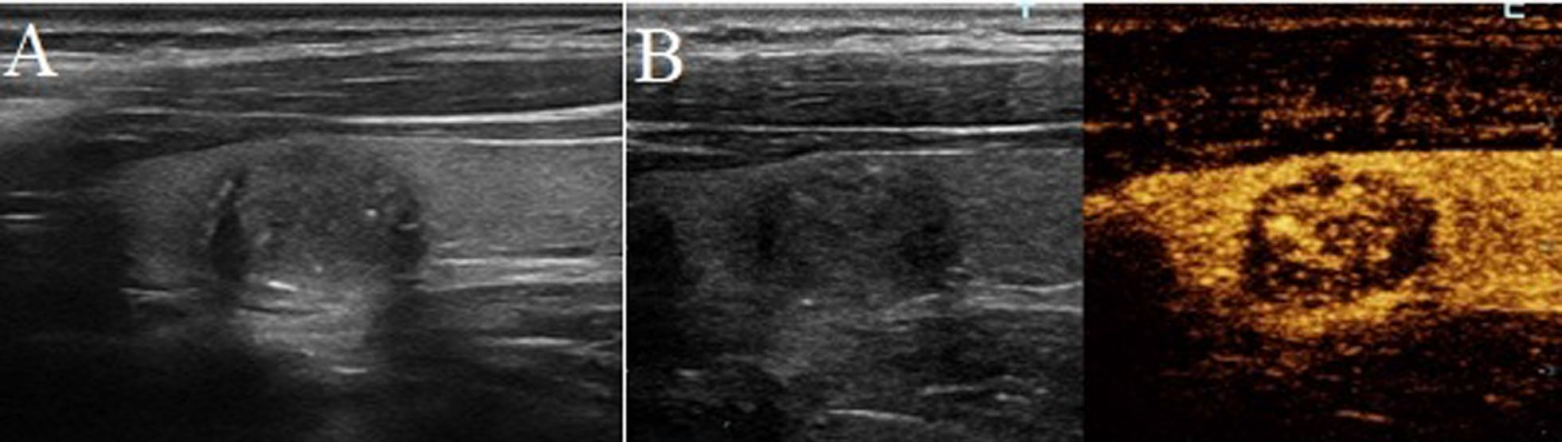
A papillary thyroid carcinoma in a 40-year-old woman. A Greyscale ultrasound showed that there was a halo surrounding the thyroid nodule. B Contrast-enhanced ultrasound revealed hypo-enhancement at peak, with a irregular low-enhancing ring (at the 20th second after the injection of contrast agent)
